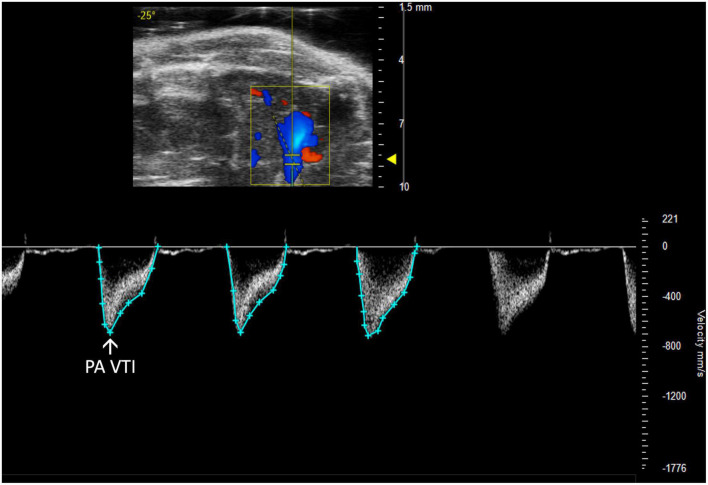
Pulmonary artery velocity time integral (PA VTI) directly measures blood flow through the pulmonary artery and is acquired as the area under the curve in pulsed wave (PW) Doppler mode of the parasternal long axis (PLAX) view.
